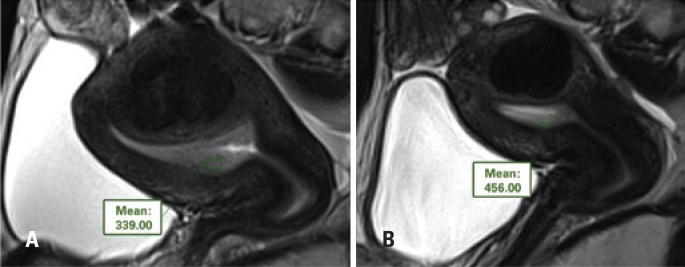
T2-weighted sagittal images demonstrating evaluation of the endometrial signal by ROIs before (A) and after (B) embolization of uterine arteries, with increased signal in (B)
